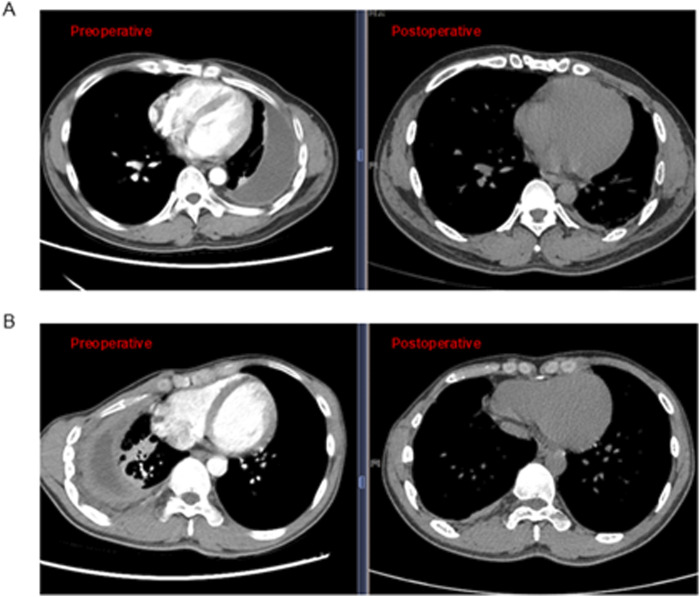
Representative chest radiographs before and after pleural decortication in patients treated with thoracotomy and uniportal video-assisted thoracoscopic surgery (VATS). A: Preoperative and postoperative radiographs from a representative patient undergoing thoracotomy. B: Preoperative and postoperative radiographs from a representative patient undergoing uniportal VATS.
